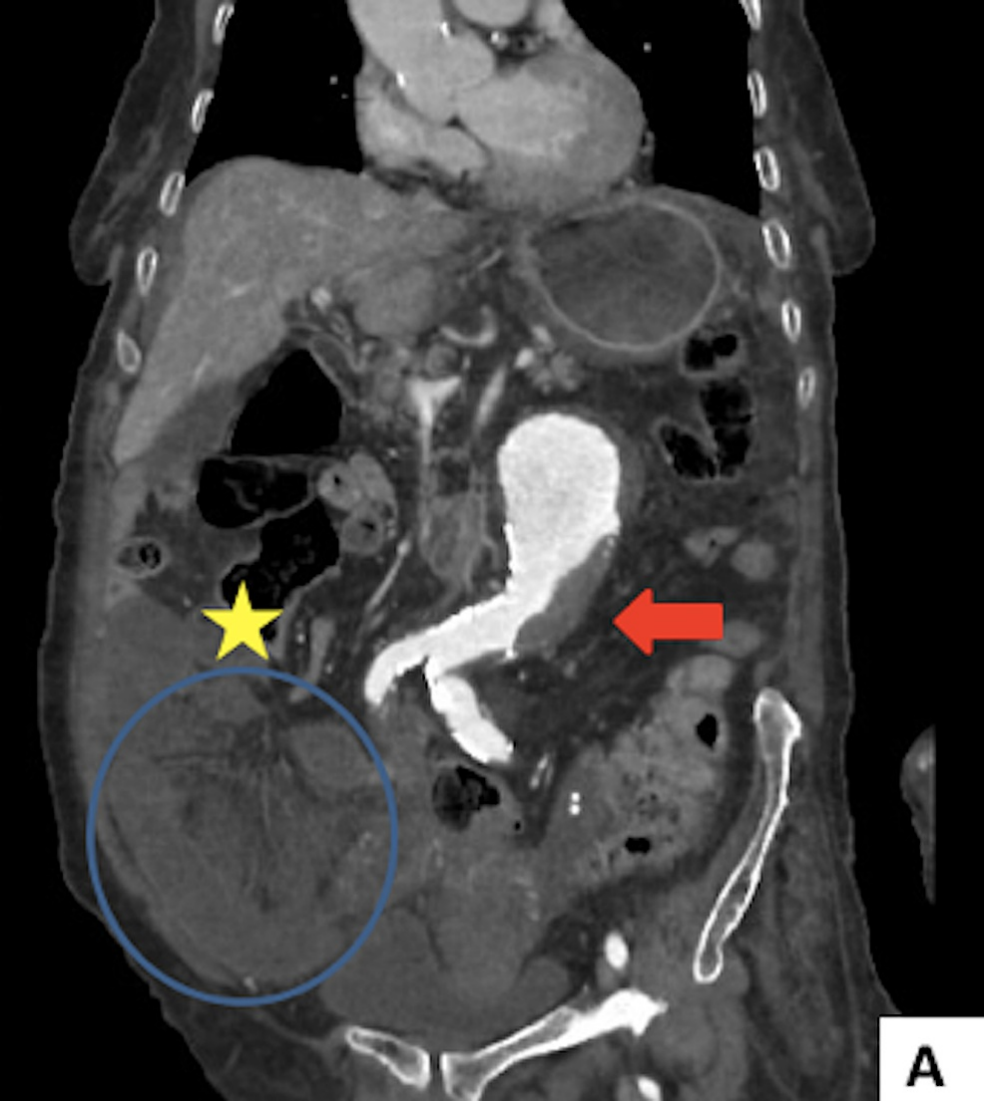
Coronal (A) CT scans demonstrating strangulated small-bowel loops in a “sac-like” formation (blue circle), shifting the ascending colon (yellow star) upward and thombosed abdominal aorta aneurysm (red arrow).
